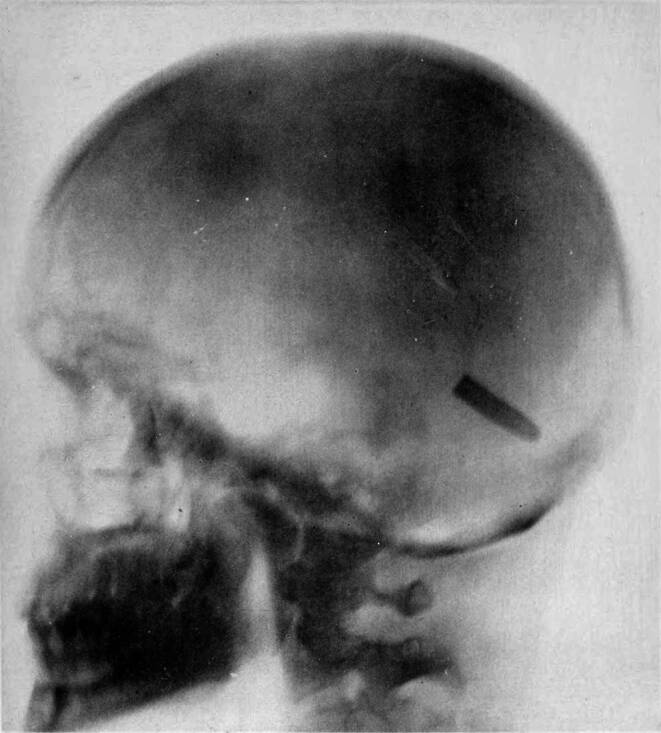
Radiograph by Elizabeth Fleischman (taken August 20, 1899) of the skull of Private John Gretzer Jr. with a bullet lodged in his brain in the region of the left occipital lobe [37]. (Public domain)
